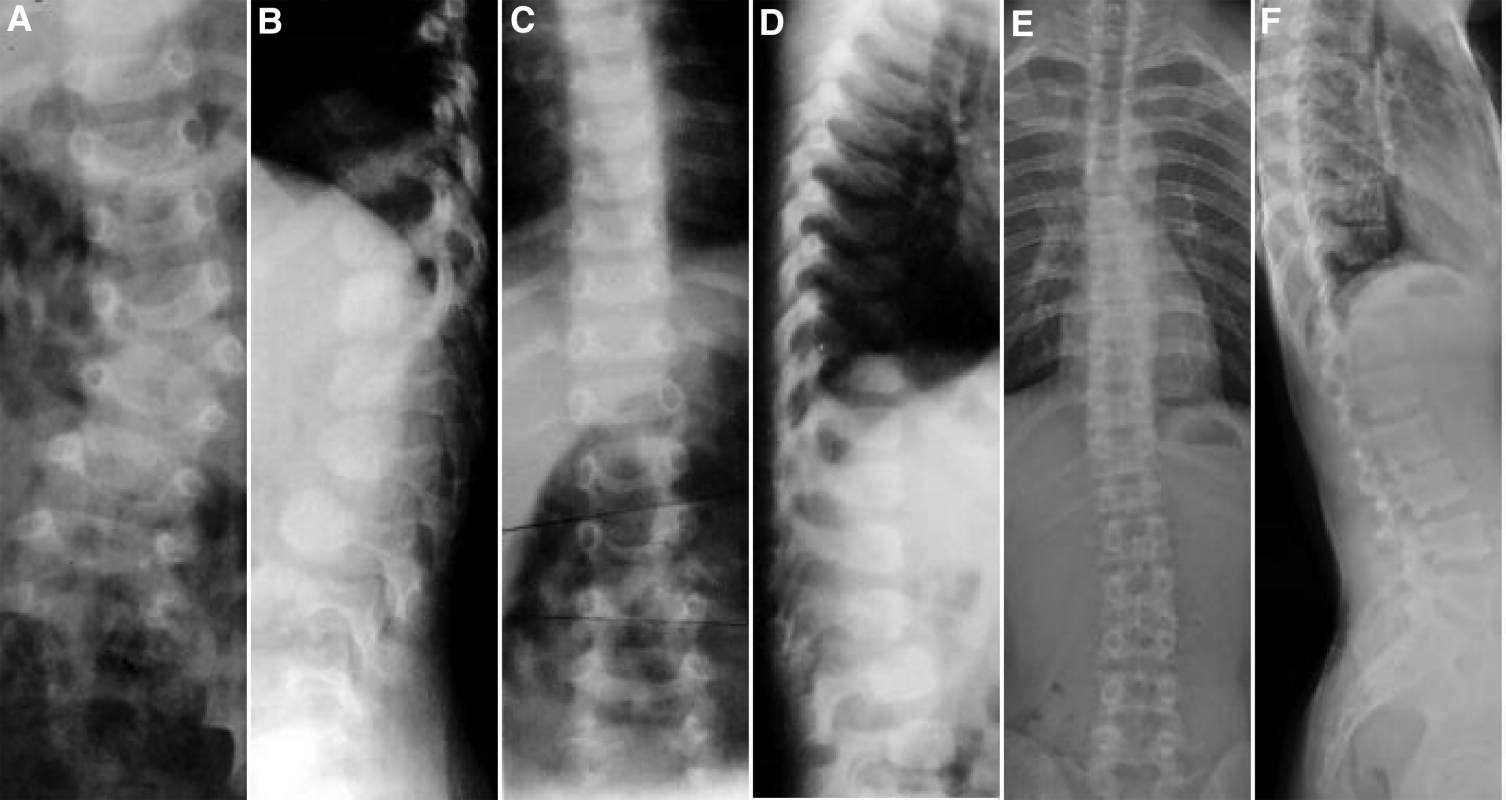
Case 8, Male, 12 months old. Preoperative orthogonal (A) and lateral (B) x-ray images showing the left hemivertebrae of L3. Intraoperative hemivertebral resection was performed *via* the posterior approach, and postoperative orthogonal (C) and lateral (D) x-rays are shown. At the follow-up at 15 years and 11 months, the orthogonal (E) and lateral (F) x-rays showed satisfactory correction of the coronal and sagittal deformity with no loss of curve correction.
